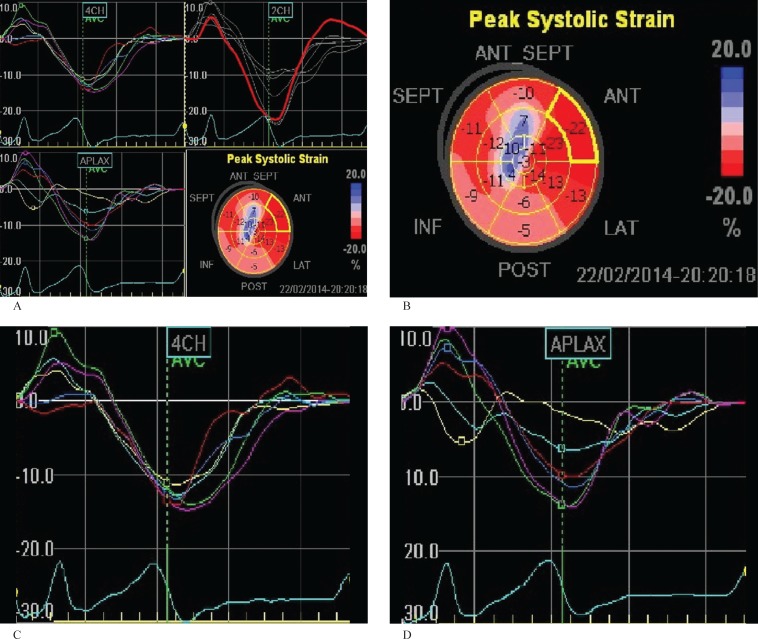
Bull’s eye and longitudinal strain curves in the four-chamber, two-chamber, and apical long-axis views in two*-*dimensional speckle tracking echocardiography*.*
